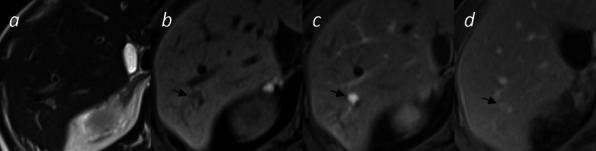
Axial MRI with T2 weighting (a), T1 fat suppression before (b), and after contrast at arterial phase (c) delayed phase—5 min (d). T2 hypersignal, T1 hypo signal, and prompt enhancement confirmed the diagnosis of the flash filled haemangioma in the hepatic segment VI (black arrows)
